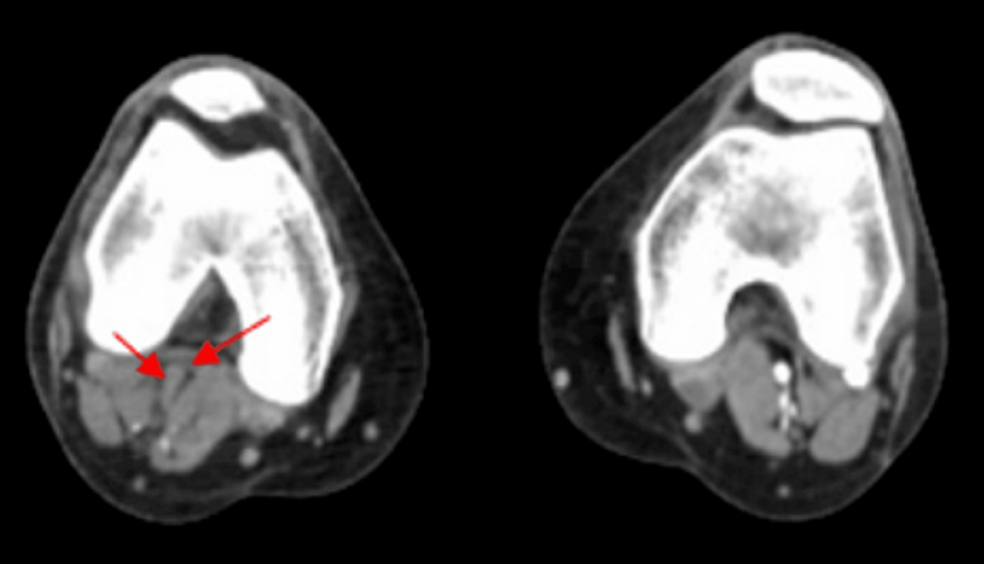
CT angiogram showed distal occlusion of the right popliteal artery.
